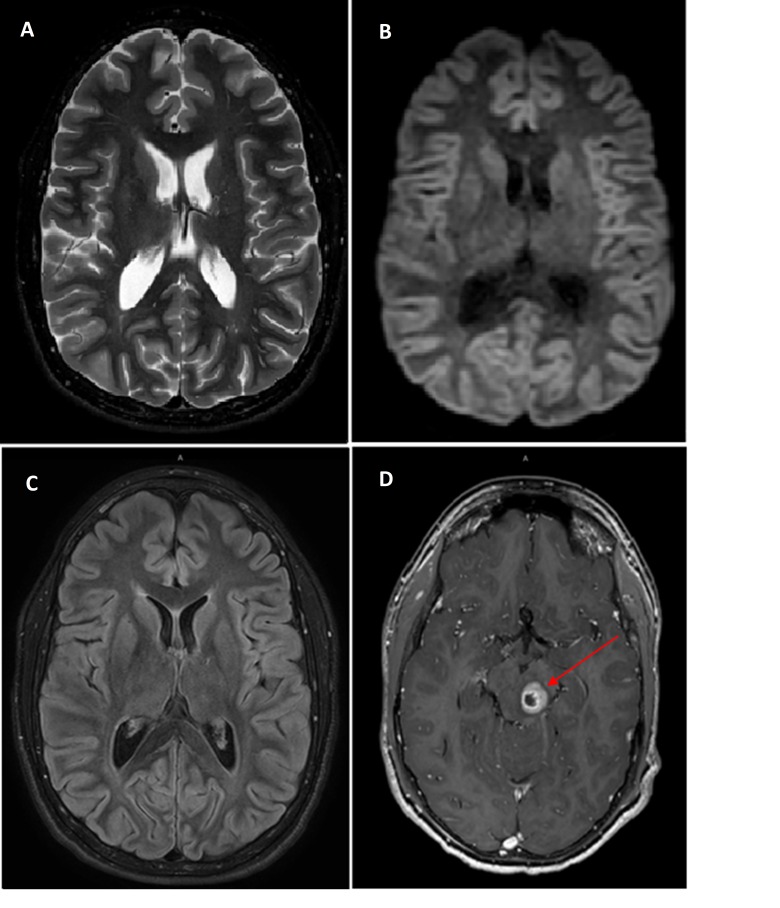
Axial T2 (A), Axial diffusion (B), Axial FLAIR (C) and Post contrast T1 images (D). Post contrast T1 image was obtained after injection of 11.5 ml of gadoterate meglumine (Dotarem) 15-year-old with Moyamoya syndrome and neurofibromatosis type-1 Findings: Magnetic resonance imaging (MRI) sequence demonstrates no acute infraction or ischemia in the brain. Note the absence of “ivy sign” on fluid-attenuated inversion recovery (FLAIR) image. Post contrast T1 image (D) demonstrates a peripherally enhancing mass in left side of midbrain, consistent with a brainstem glioma (red arrow)
